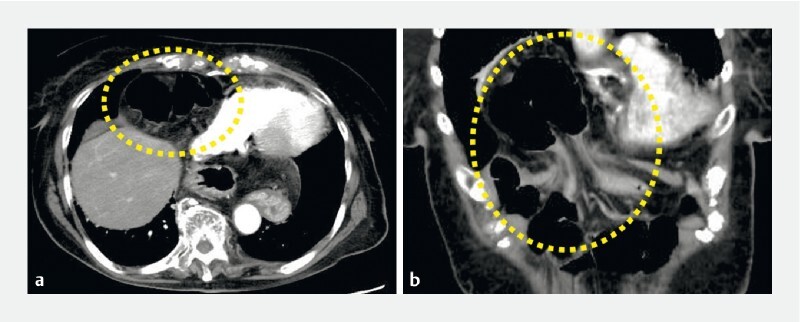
A computed tomography scan showed prolapse of the transverse colon into the mediastinum and was consistent with obstruction due to a Morgagni hernia.
